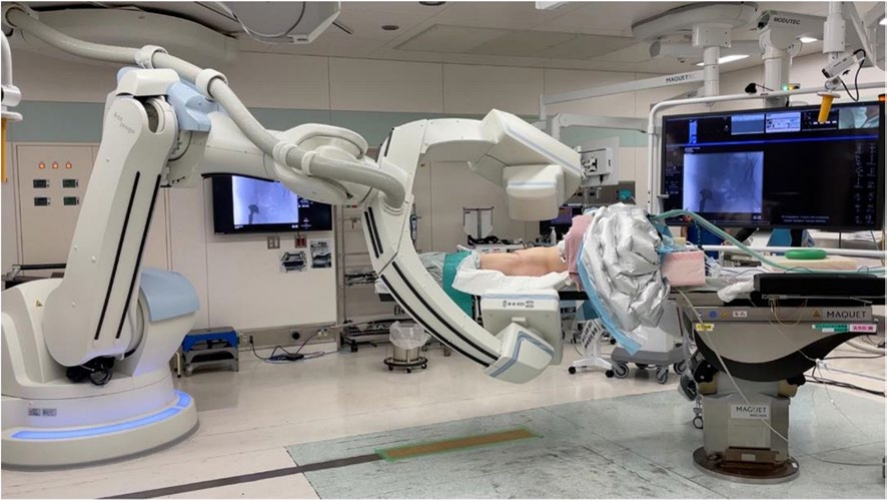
Hybrid operating room equipped with a 3D flat-panel C-arm (Artis Zeego, Siemens). Surgery was performed in the supine position and intraoperative C-arm CBCT was performed under general anesthesia
